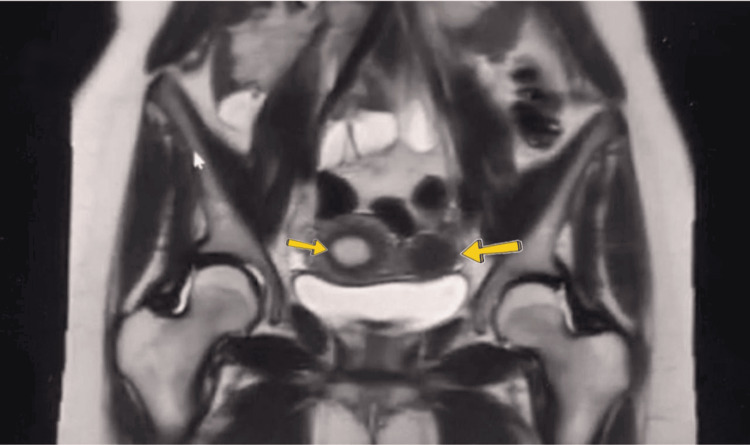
Pelvic MRI revealing both tubes and ovaries connected to the unicornuate uterus (right arrow) and the presence of a non-communicating rudimentary horn (left arrow). MRI: Magnetic resonance imaging
